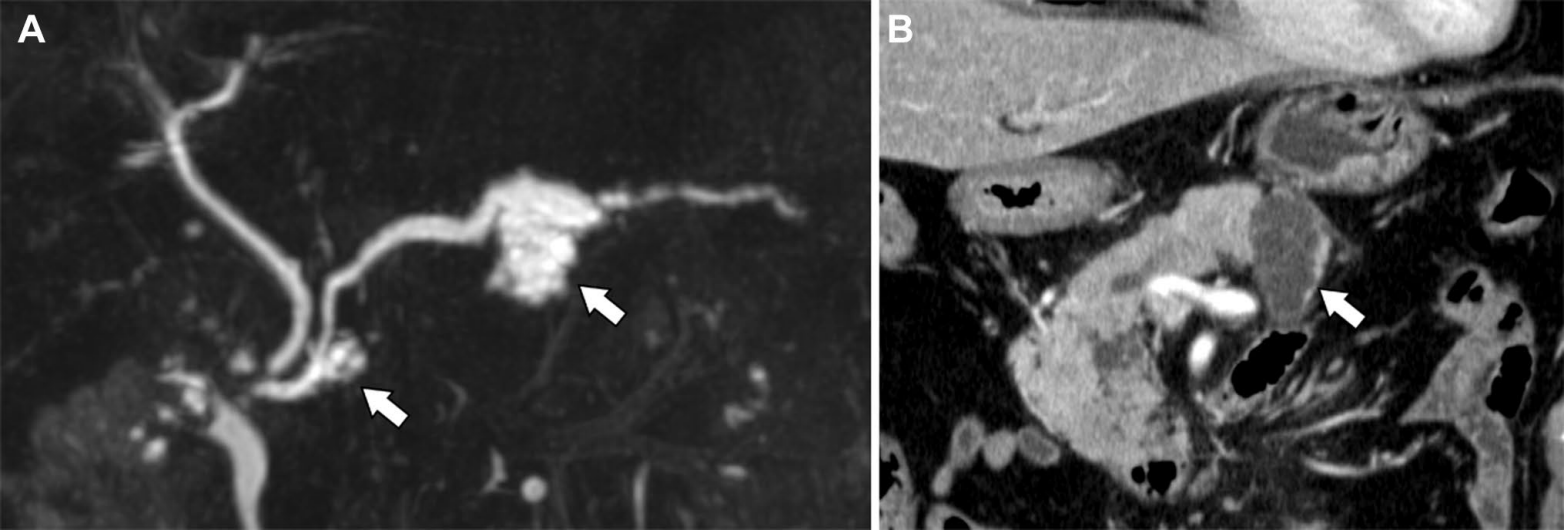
Case of a 74-year-old man suffering from recurrent pancreatitis. Magnetic resonance cholangiopancreatography shows multilocular cystic mass in the head and body of the pancreas (a: arrow). Main pancreatic duct is dilated without obstruction. On coronal contrast-enhanced computed tomography, cystic lesion in the body of the pancreas has no solid component (b: arrow). Both were resected and pathologically diagnosed as intraductal papillary mucinous adenoma.
